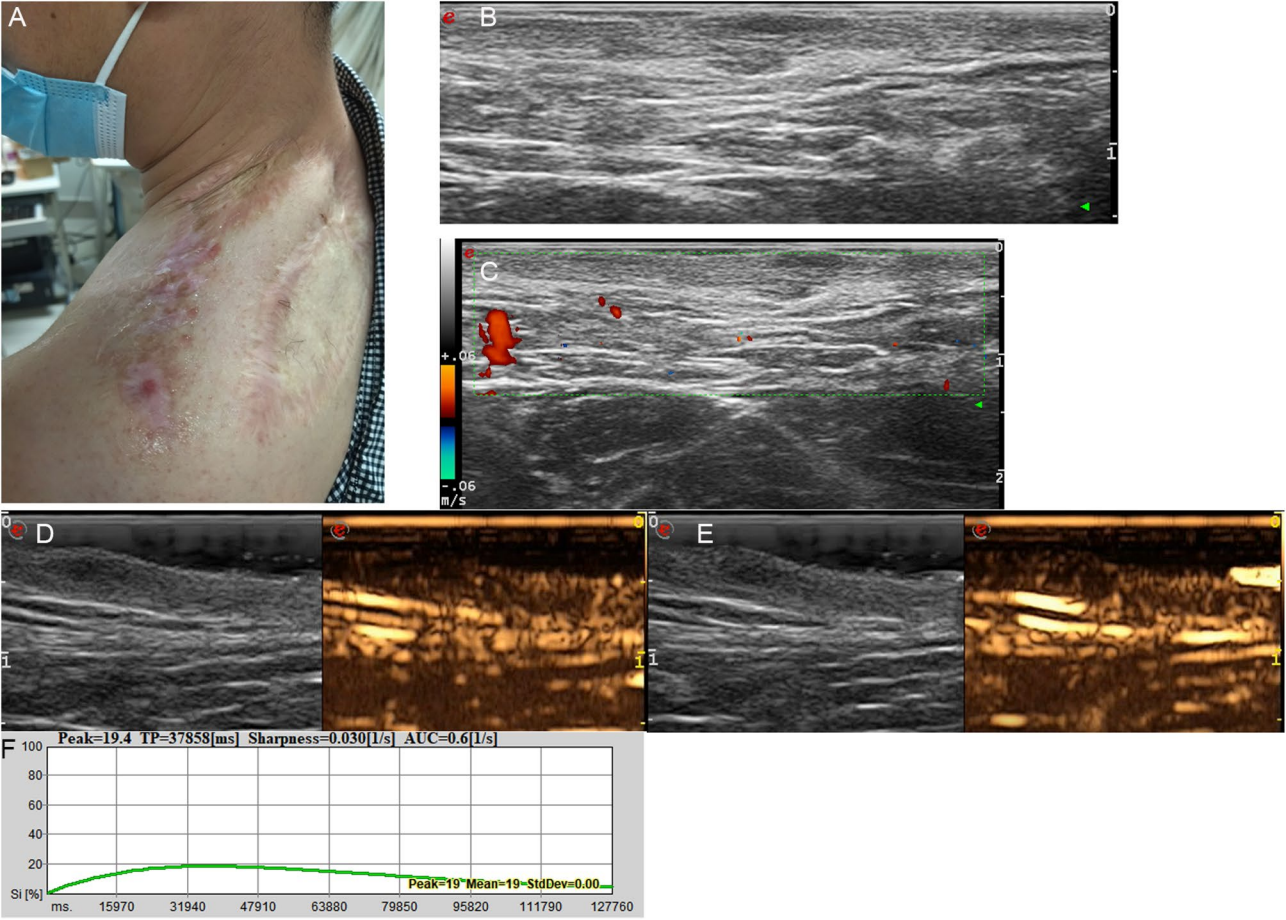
A Post-resection scar with red protrusion in the left shoulder of a 46-year-old man. B Transverse ultrasonogram revealing a well-defined, hypoechoic, homogeneous, and subcutaneous lesion. C Color Doppler ultrasonogram revealing no blood flow signal. D Contrast-enhanced ultrasound revealed the trend that contrast agent enters the lesion from the bottom. E Contrast-enhanced ultrasound revealed homogeneous hyper-enhancement at peak (the 38th second). F Time intensity curve revealed peak of 19.4 and sharpness of 0.030
